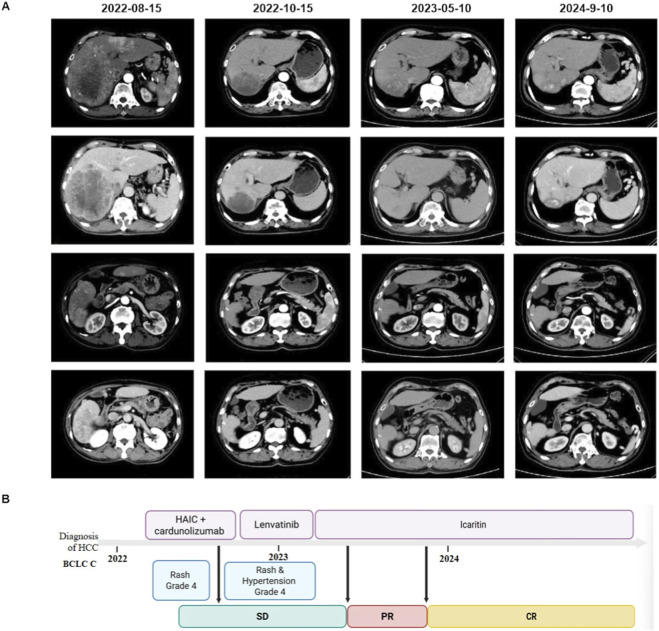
A representative patient treated with icaritin monotherapy. (A) CT images of the patient at initial diagnosis and during icaritin treatment. (B) Treatment process of patients.
